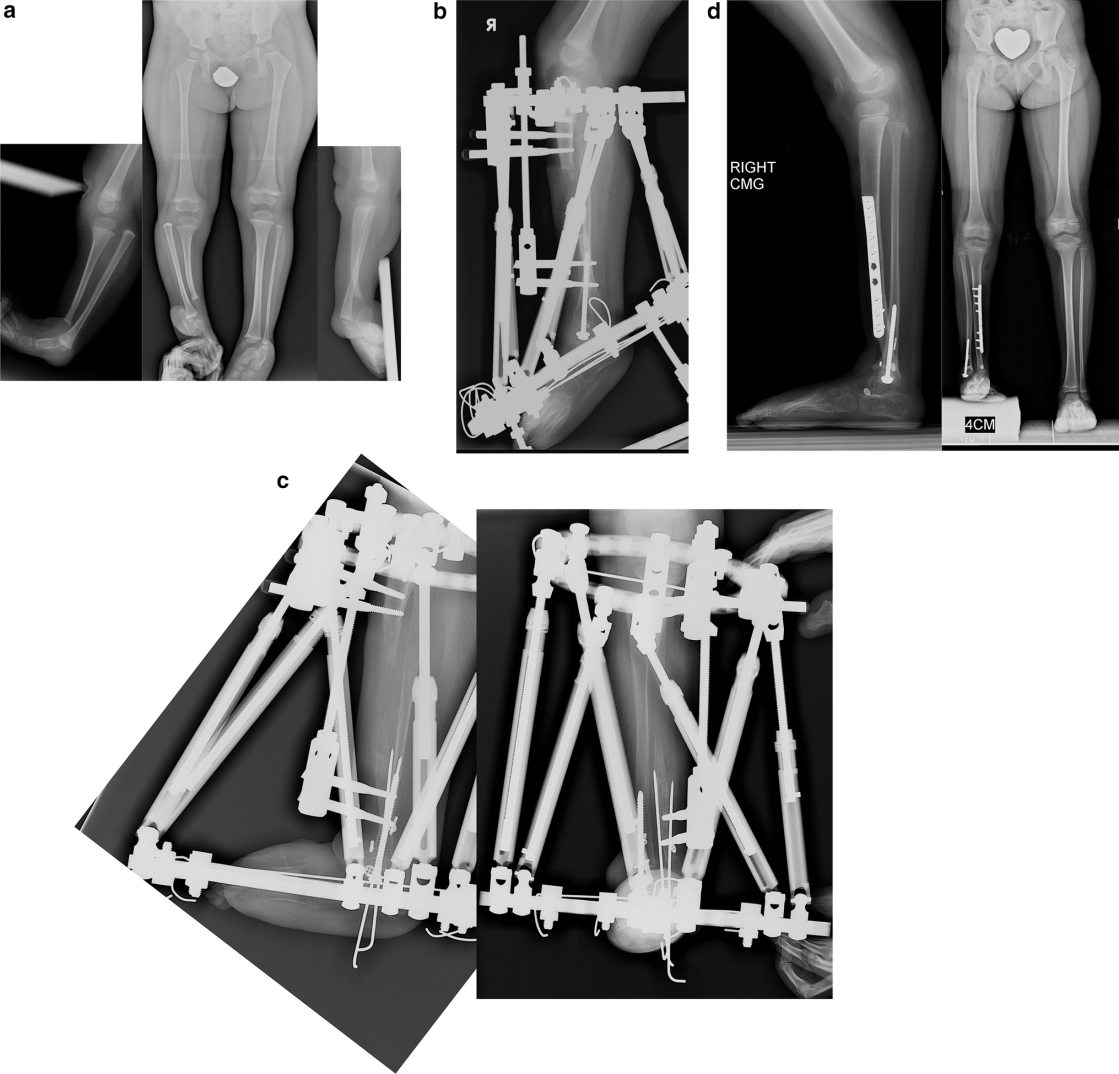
a Radiographs of 2-year-old girl with Paley type 2A unilateral tibial hemimelia, with equino-varus deformity of foot and varus of tibial diaphysis. There is no diastasis of the distal tibio-fibular joint. The ankle joint is present. The ossification of the distal tibia shows a regular trumpet shaped metaphyseal line indicating that this is the region of the distal tibial physis. b Lateral radiograph of tibia. Taylor spatial frame (TSF) is in place with proximal tibial and foot rings programmed for gradual correction of foot deformity. There is also an independent mechanism (threaded rod with cube and two half pins connected to the proximal ring) to simultaneously lengthen the tibia through a proximal osteotomy. There is a distal fibular epiphysiodesis screw in place to slow the growth of the faster growing fibula. c Lateral (*left*) and AP (*right*) radiographs at the end of the foot correction and 8 cm of lengthening after a second stage surgery to openly reduce the talus to the tibia combined with axial pinning of the ankle joint. Syndesmotic washer-suture device (Ziptite™ Fixation System; Biomet Sports Medicine, LLC, Warsaw, IN) inserted to stabilize the lengthened tibia to the fibula at its new syndesmosis level. The tibial lengthening bone shows poor regenerate formation. d Plate fixation at time of removal of fixator to prevent fracture. The tibia is well aligned. The talus is under the distal tibia. The fibula is now at station at the ankle and the ankle is stable. The foot is plantigrade
